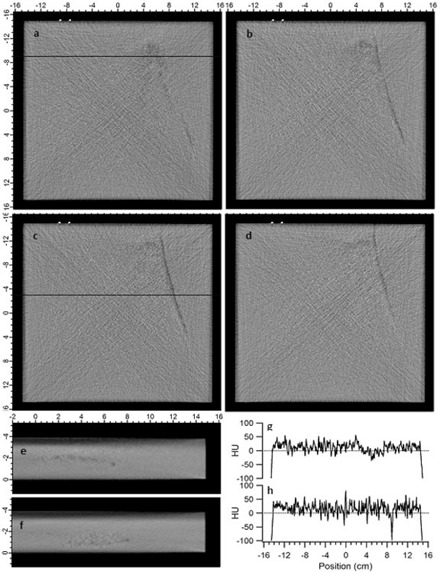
CT images of Sample A showing representative density variations (a)–(d) in the plane of the slab, and in the cross section (e) and (f) at the location indicated by the horizontal lines in (a) and (c), respectively. Line profiles (g) and (h) are shown from (a) and (c), respectively, as indicated by the horizontal lines.
